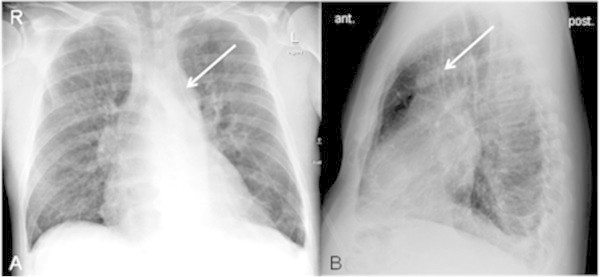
CVC in chest X-ray. A: Anterior to posterior chest X-ray with a CVC installation (arrow) in the persistent left superior vena cava detected (lying patient). B: lateral view showing the catheter (standing patient).
